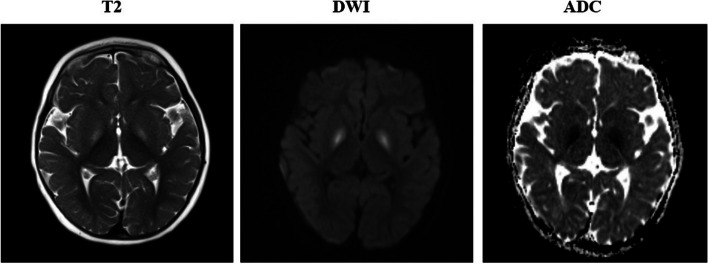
MRI at presentation age 12 months, T2 sequences demonstrating increased signal of the globus pallidus bilaterally. DWI and ADC demonstrating bilateral diffusion restriction in the globus pallidus
